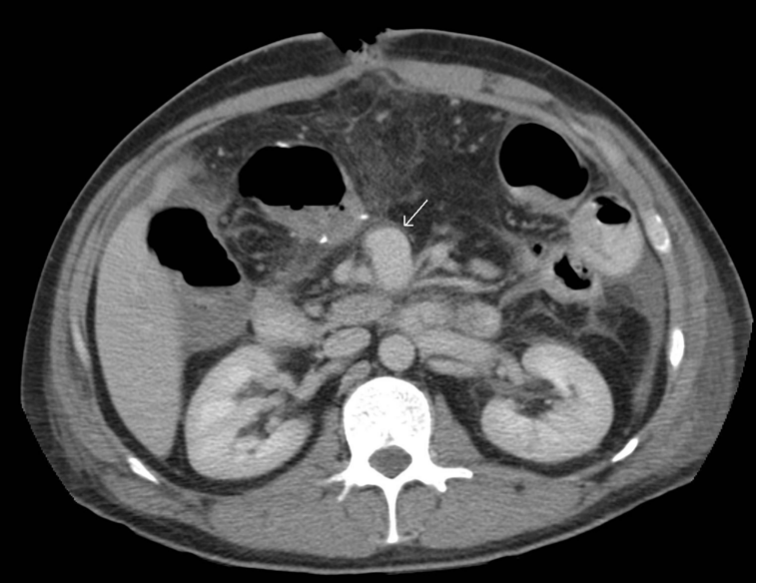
Axial CT image with oral contrast demonstrating a small pseudoaneurysm (arrow) to the right of the SMA.
